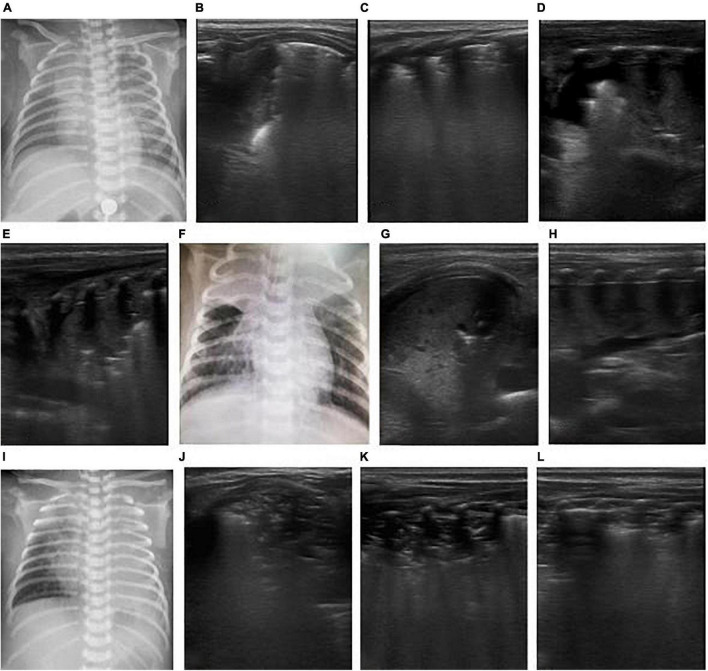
The patient was a 26-day-old, full-term neonate and admitted to the hospital due to cough and shortness of breath for 4 days and aggravation for 2 days. The neonate had a spasmodic cough, and his mother had a history of cough. The nucleic acid test of respiratory virus suggested pertussis. It was diagnosed as severe pertussis pneumonia. On the admission day, LUS showed bilateral consolidation with atelectasis in the right upper lung (B,C), and chest X-ray showed consolidation of the right upper lung (A). After admission, the patient was given anti-infection and CPAP respiratory support, but after half a day, the respiratory distress progressively worsened, and blood oxygen decreased. Re-examination by LUS showed increased consolidation of both lungs as a hepatoid echo and developed to a large area atelectasis. Bronchial inflation sign was reduced, and consolidation was severe (D–F). Hence, mechanical ventilation was provided *via* endotracheal intubation for respiratory support. An emergency exchange transfusion was performed. WBC increased again after 3 days of hospitalization, and atelectasis was aggravated (G–I), and exchange transfusion was performed again. After the second exchange transfusion, ultrasound monitored pulmonary atelectasis was improved (J–L).
